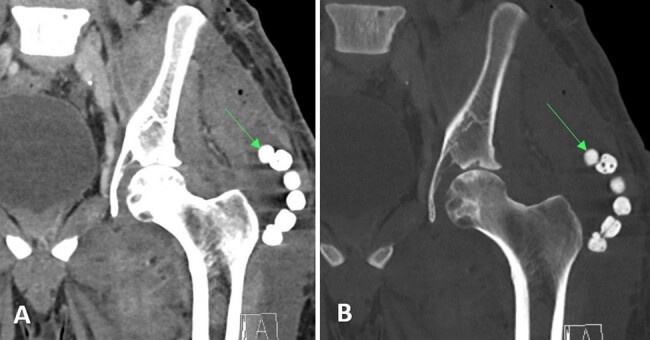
Case 1 post-op CT scan of the pelvis demonstrating antibiotic beads (arrows) in the left thigh.
